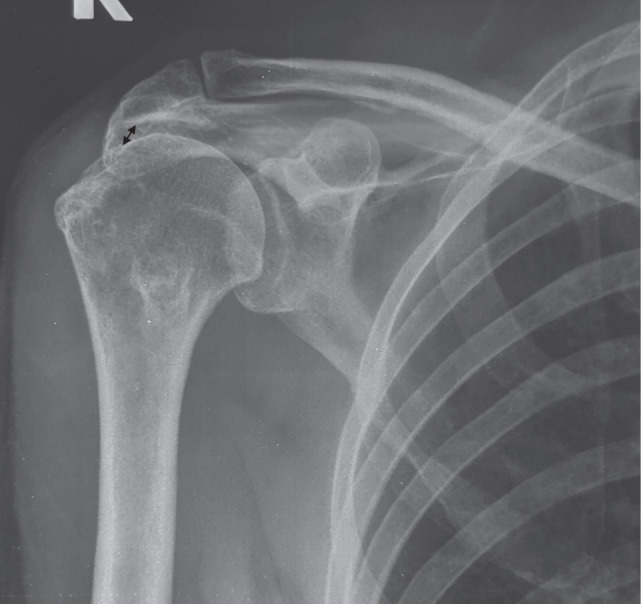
Anterior-posterior view of a shoulder radiograph showing narrowed acromio-humeral interval (arrow), which is superior migration of the humeral head indicating rotator cuff arthropathy. Intra-operatively, the rotator cuff was found irreparably destroyed.
